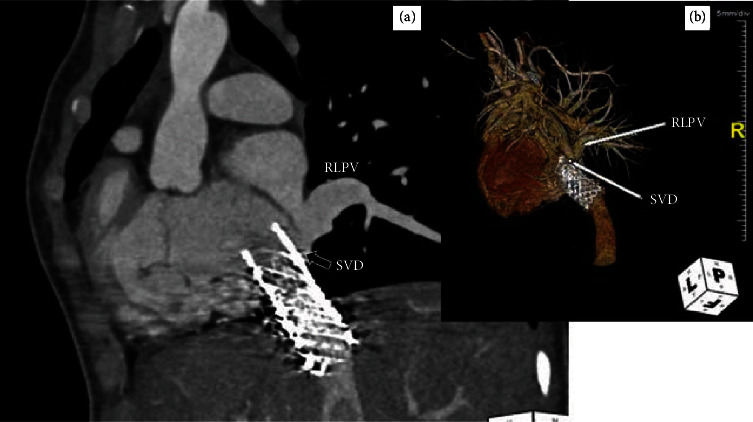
Postprocedural computed tomography angiogram showing (a) a sagittal image and (b) a rendered image of a patent 3-stent structure in the inferior vena cava. The stent structure covers the sinus venosus defect (SVD) with the upper end almost opposed to the interatrial septum causing a small residual opening (black arrow) and unobstructed blood flow from the right lower pulmonary vein (RLPV) to the left atrium (a). The superior segment of the covered stent should be flared by balloon to prevent residual shunt.
